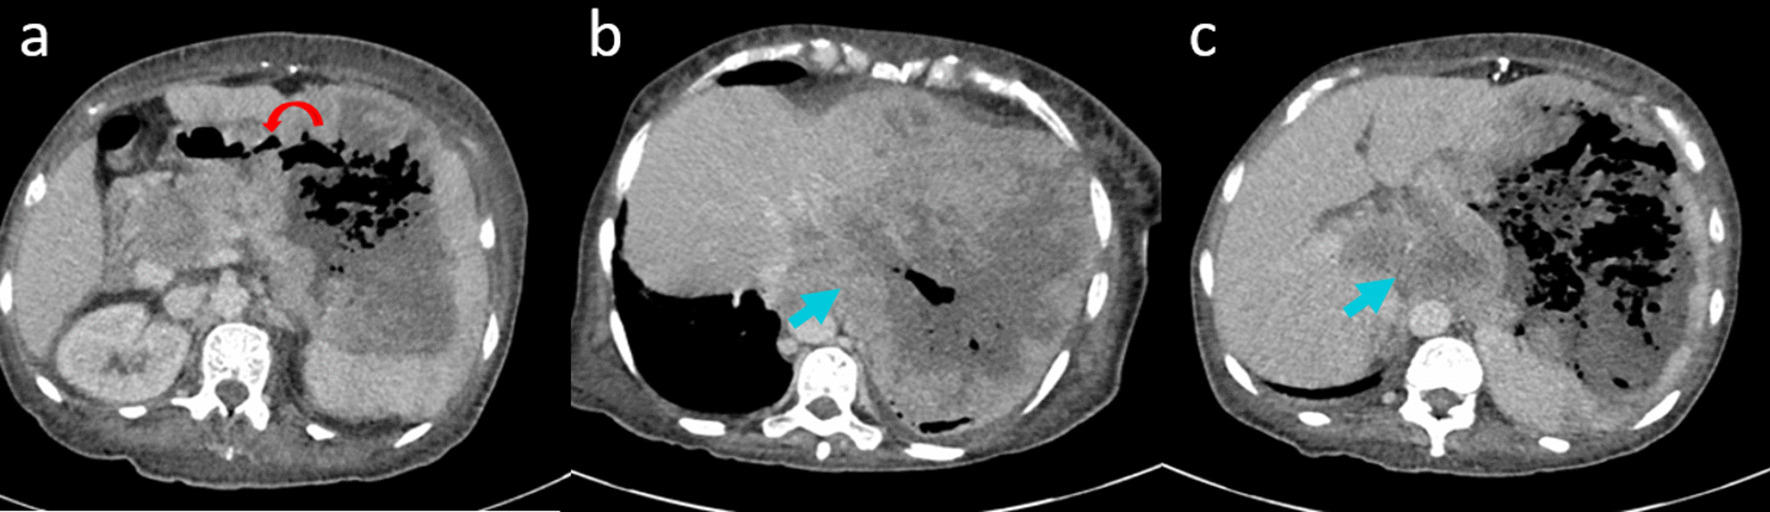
Abdominal CT scan views. a A gas-containing splenic mass due to the fistulization in the stomach (red arrow) b, c retroperitoneal lymphadenopathy (blue arrow)
